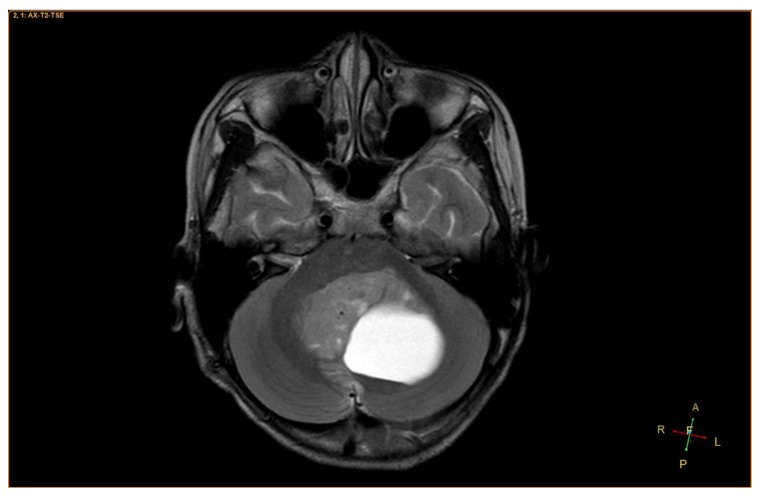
A heterogeneous mass, located in the fourth ventricle, containing both solid and cystic components, on axial T2-weighted imaging.
